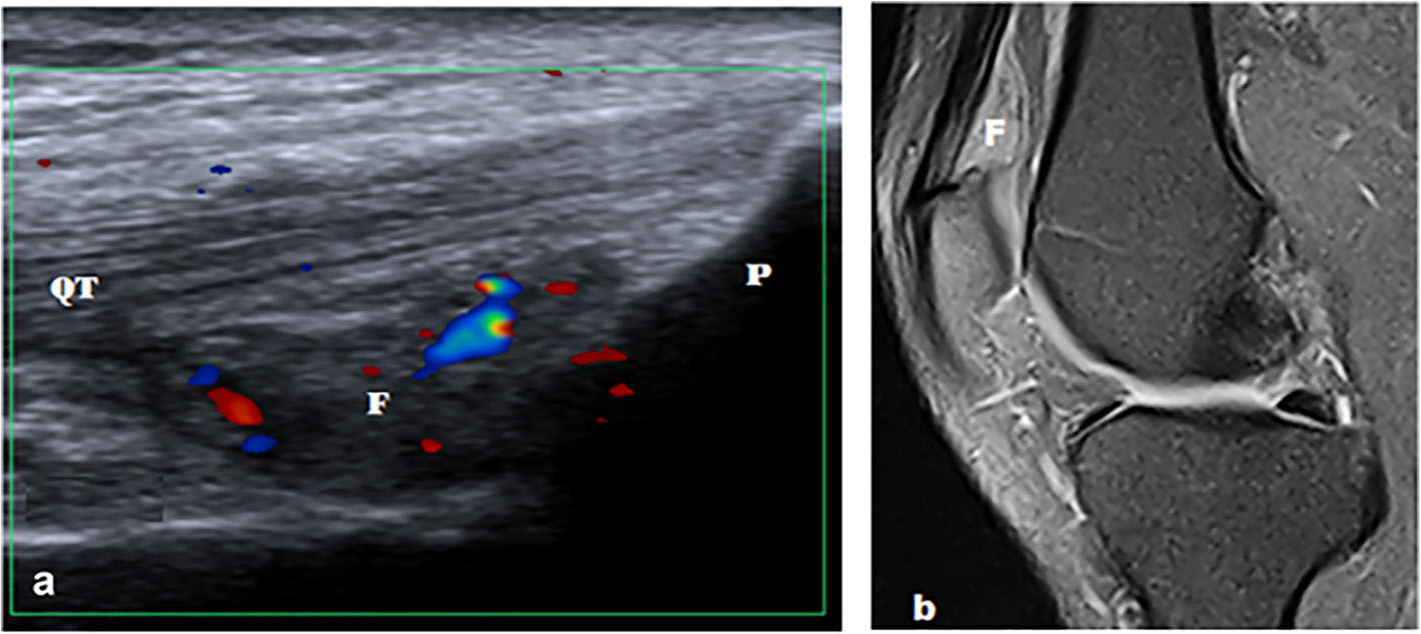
A 37-year-old male with left suprapatellar pad of fat impingement. a Long axis ultrasound image shows hypoechoic suprapatellar fat pad with convex posterior margin. Color Doppler ultrasound shows increased vascularity. b Sagittal PDFS MR image shows hyperintense suprapatellar fat pad with convex posterior margin. QT, quadriceps tendon; P, patella; F, suprapatellar fat pad
